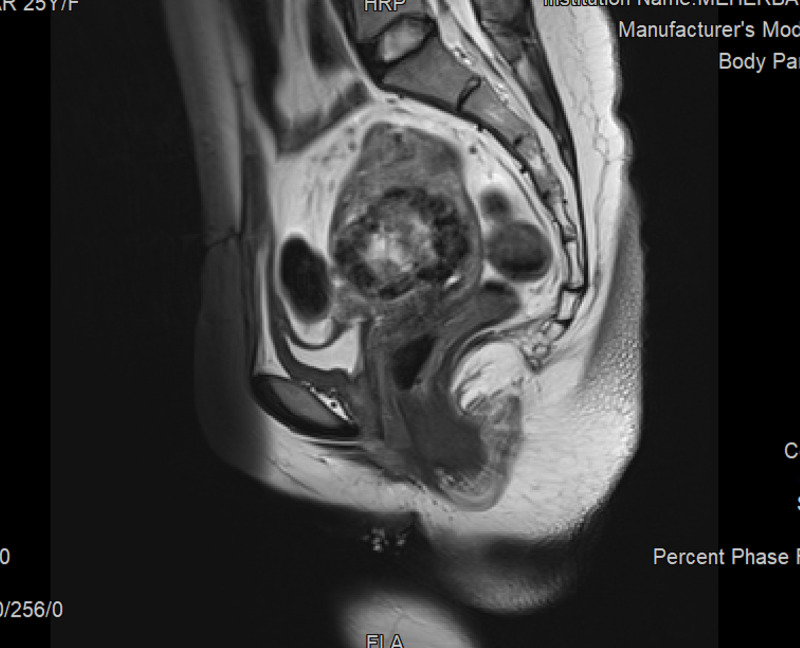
MRI report of Case 1 , showing scar ectopic pregnancy. The Type 2 scar ectopic is seen eroding the scar anteriorly which was seen as bulging scar intraoperatively. This was diagnosed as invasive mole by radiologists for the lack of definite gestational sac.
